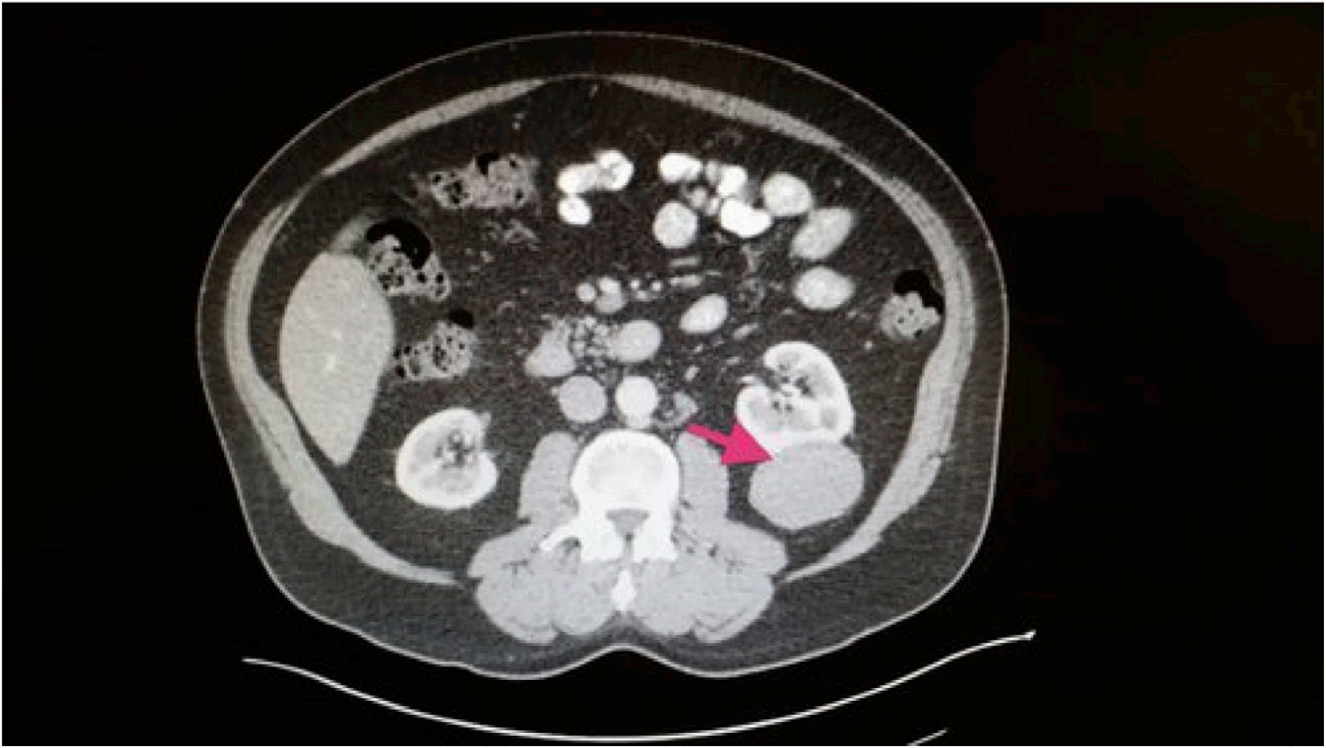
Computed Tomography Image of Patient BP's Renal Cell Carcinoma Imaged in February of 2014, this thoracic computed tomogram with intravenous contrast shows a 5.7 × 4.8-cm mass in the left kidney.
